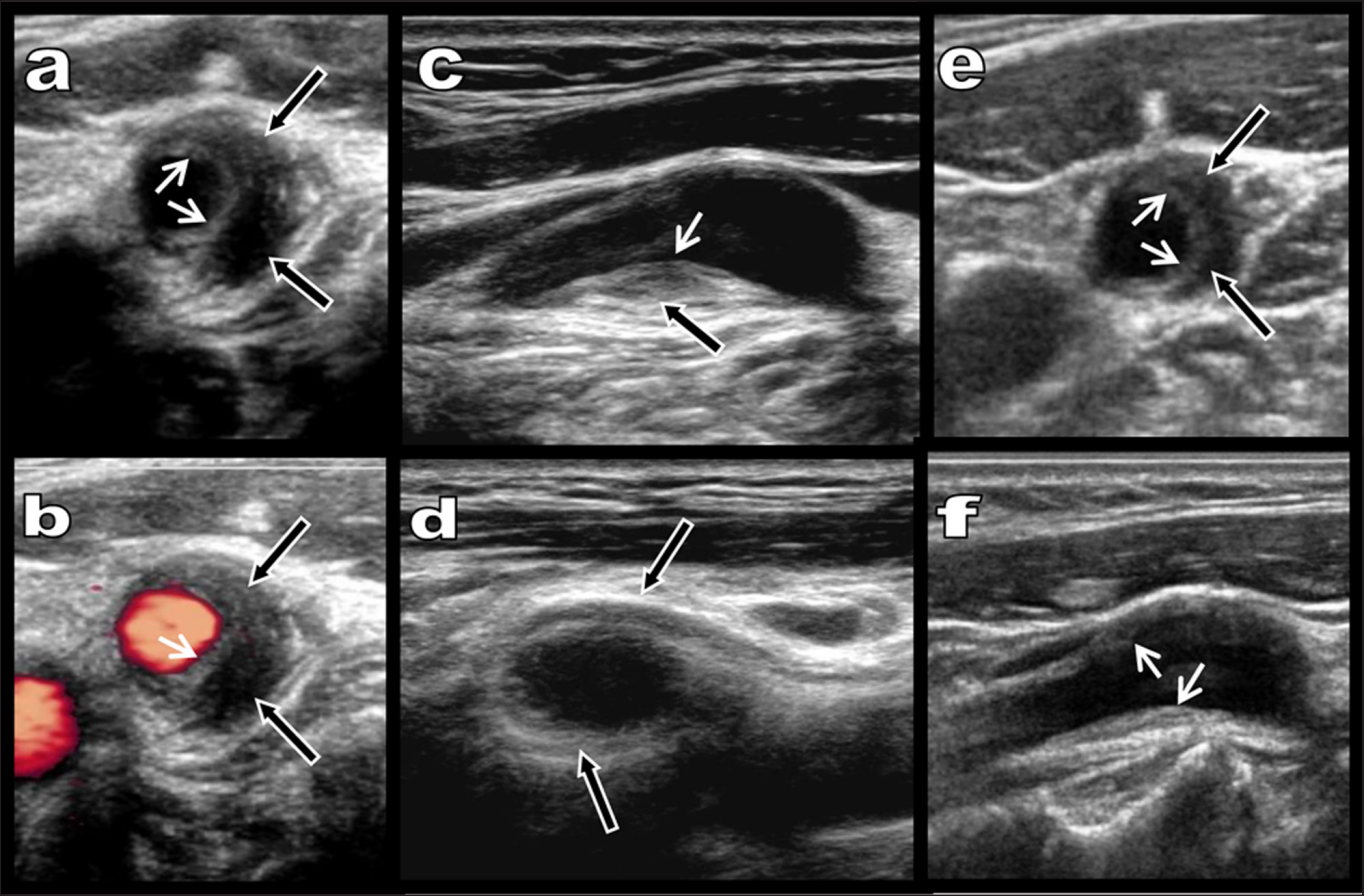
Grayscale (a and c–f) and Colour Doppler ultrasound (b) show a massive eccentric and preferentially laterally developed hypoechoic adventitial thickening of the bulbar internal carotid (black arrows). A thin hyperechoic intimal fibrous plaque is seen (small white arrows). Stenosis is absent. Drastic regression of the hypoechoic thickening is already found after only 15 days of treatment with non steroidal anti-inflammatory drugs (e and f). The thin hyperchoic intimal plaque persists.
